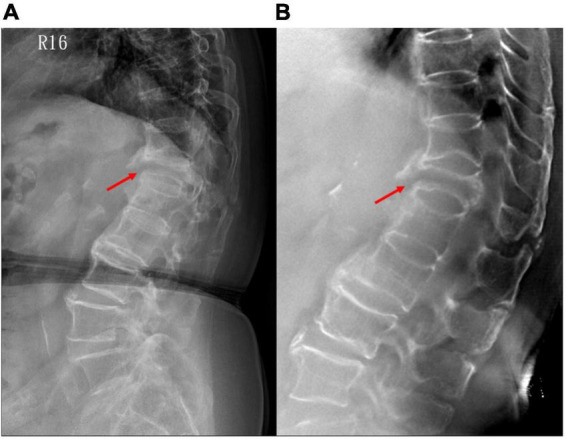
A patient with non-union of VCF on the 3-month follow-up. (A) Plain radiograph shows non-union with a gap at the anterior vertebral edge (arrow); while (B) tomosynthesis more clearly demonstrates both anterior and posterior vertebral edge involvement. VCF, vertebral compression fracture.
